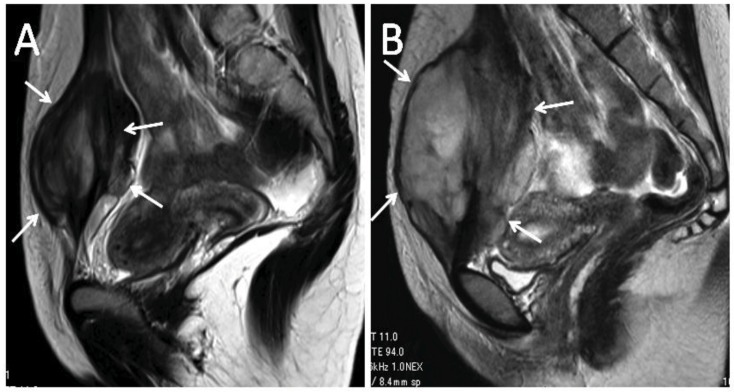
Twenty years old female with desmoid (arrows) in abdominal rectus muscle. (A) T2-weighed sagittal image of MRI before meloxicam treatment. (B) MRI of the same patient after 8 months’ treatment with meloxicam. The patient was subjected to the surgical treatment.
